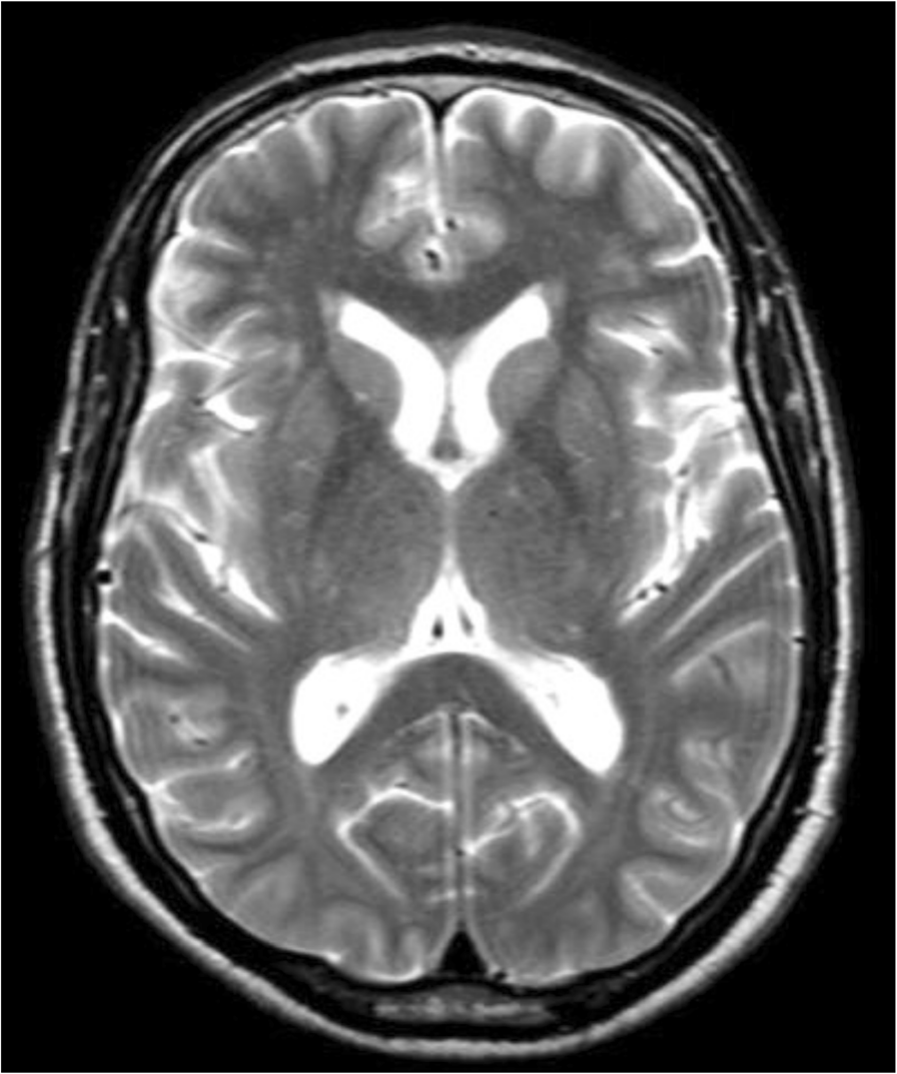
Magnetic resonance imaging of the brain: T2 image, showing no lesions
